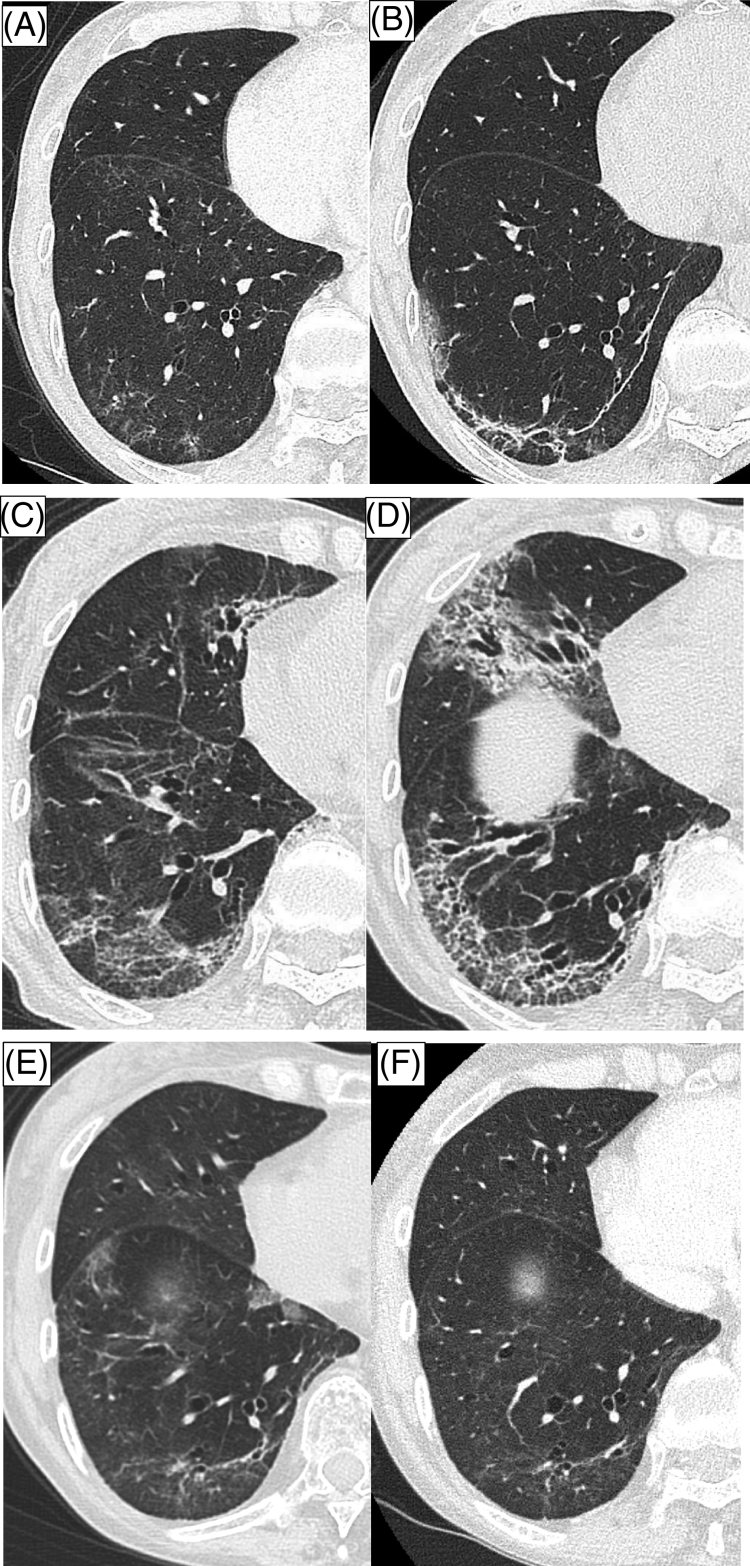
Thin‐slice computed tomography of the left lower lobe in chronological order. (A) Progressive infiltration of bilateral, patchy ground‐glass opacities (GGO) at the onset of COVID‐19. (B) New appearance of GGO after 2 months from the onset. (C) Bronchial traction appeared after the m‐PSL dosage was reduced <30 mg. (D) Progression of the GGO and reticular shadow after 8 months from the onset. (E) Improvement of the GGO after initiation of tacrolimus. (F) Resolution of reticular shadow after 14 months from the onset.
